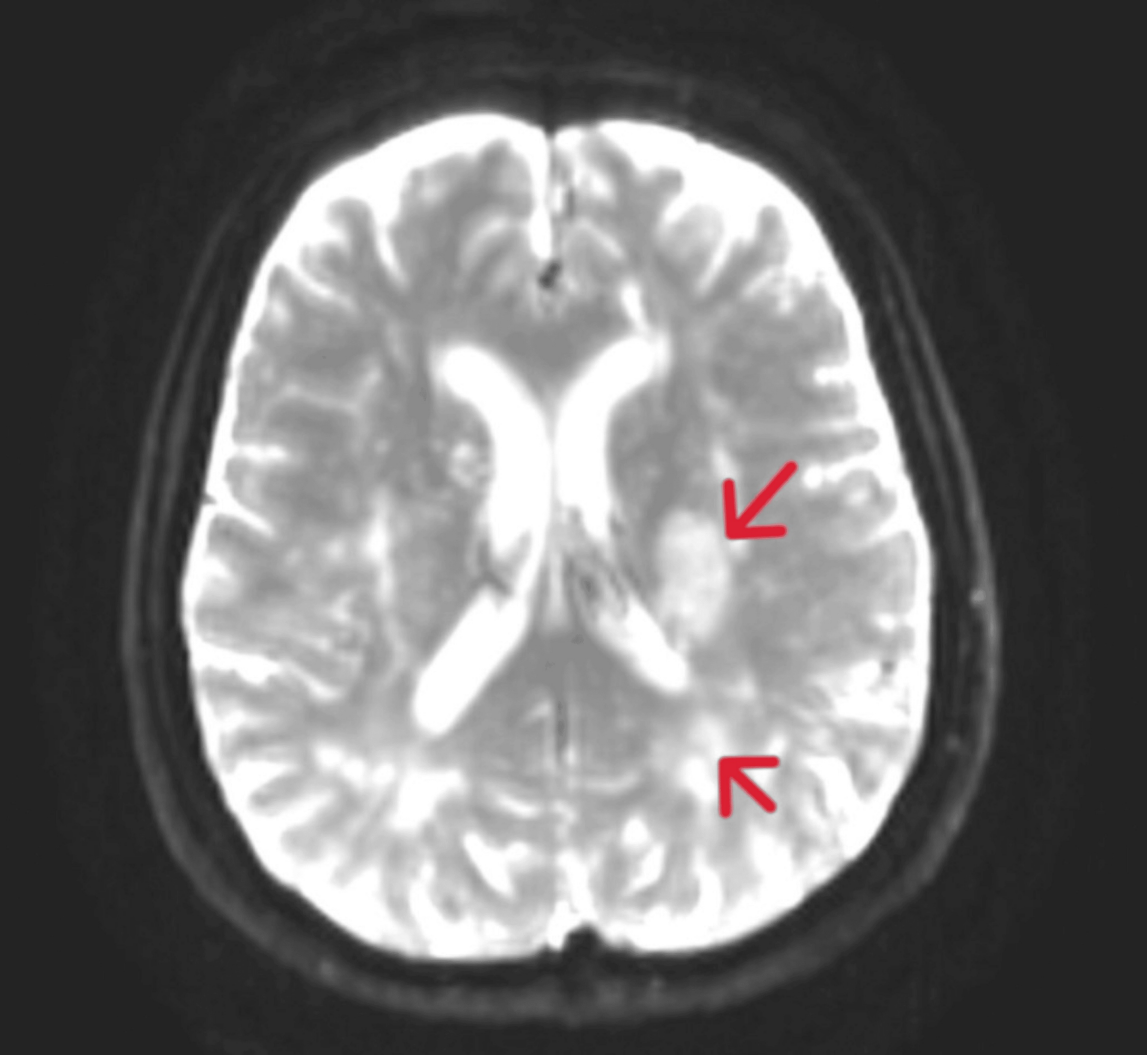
Axial view of cerebral MRI (DWI). The red arrows show the acute ischemic lesion MRI: magnetic resonance imaging; DWI: diffusion-weighted imaging
